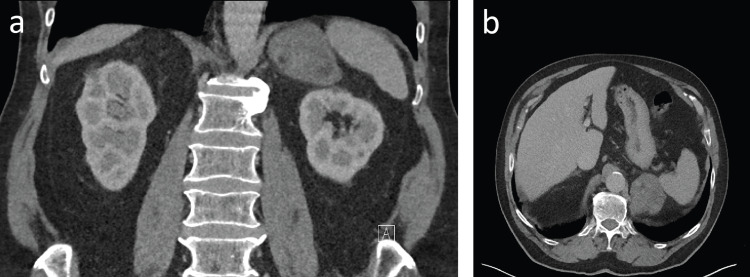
The index computed tomography scan in 2014. (a) Coronal view showing a left adrenal mass measuring 5.5 × 4.8 × 5.6 cm. (b) Axial view.
